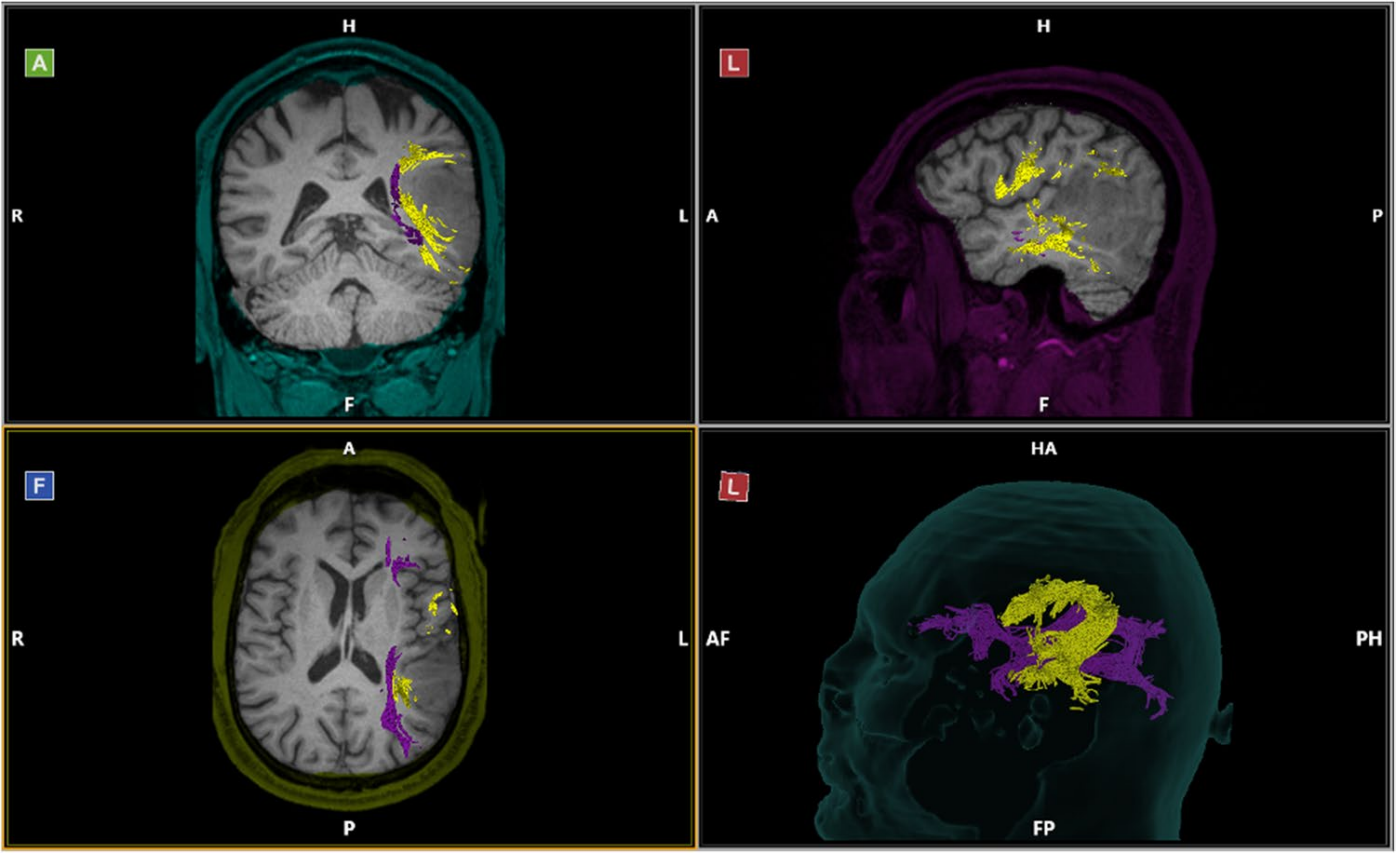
T1-weighted sequence showing a left temporal posterior non-enhancing lesion. The AF (yellow) is shown, surrounding the lesion anteriorly and medially. The IFOF (purple) is intimately related to the lesion’s inferior and medial borders
